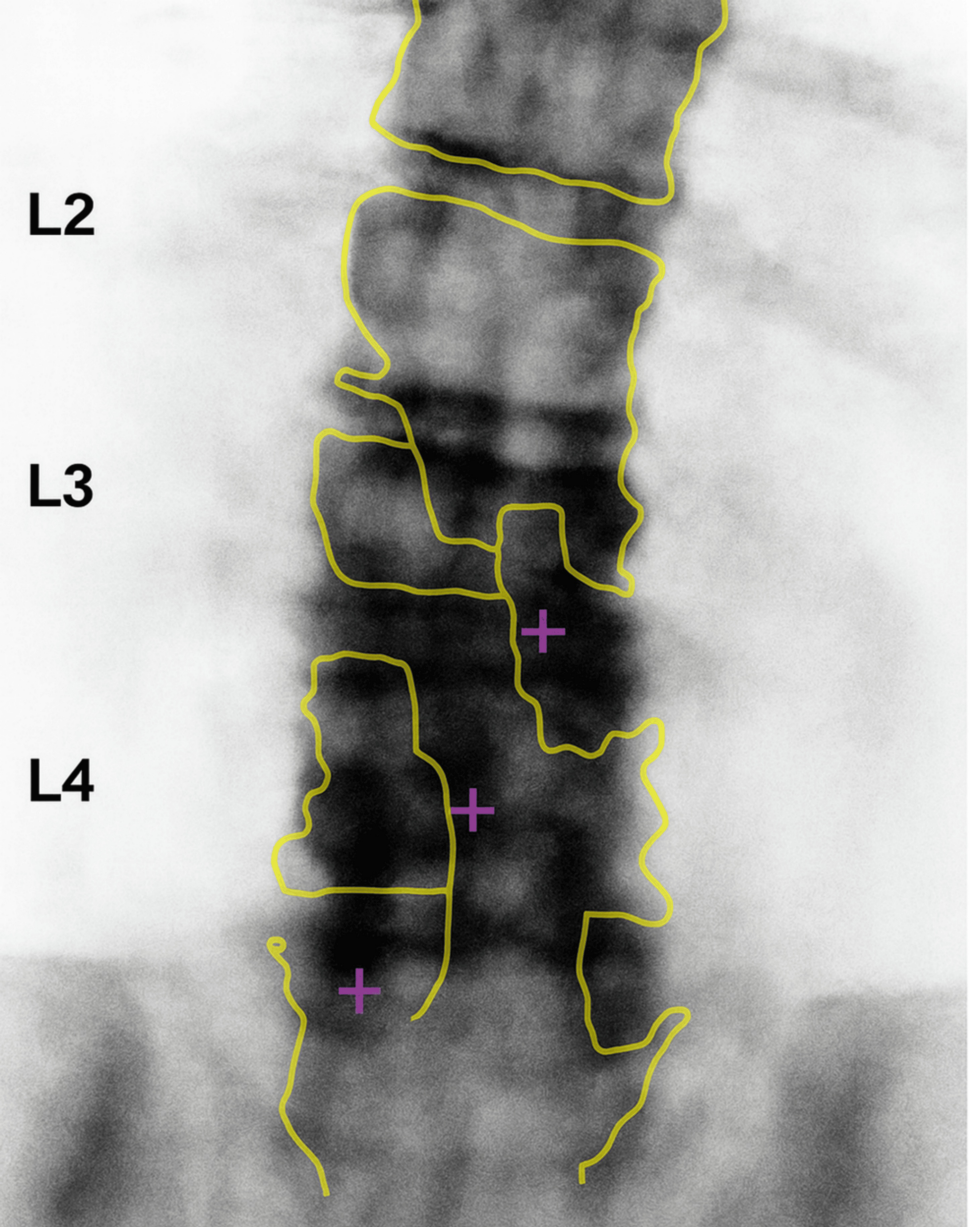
Dual-energy X-ray absorptiometry (DEXA) image of the lumbar spine (L1-L4), demonstrating reduced bone mineral density consistent with a low T-score measurement.
